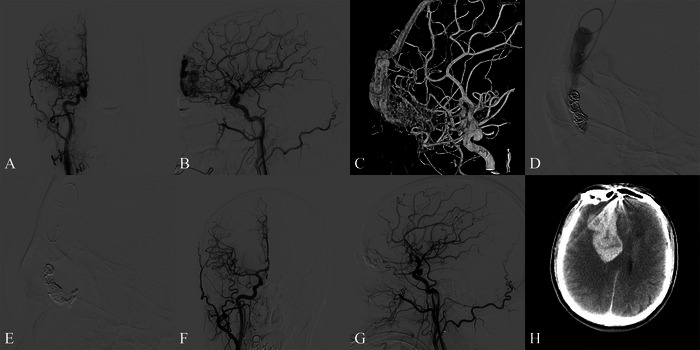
The patient was incidentally diagnosed with an intracranial vascular malformation during routine medical examination. Anteroposterior (A) and lateral (B) views of the right internal carotid artery angiogram revealed a frontal base MPD‐AVM supplied by both the anterior ethmoidal artery and the orbitofrontal artery. 3D reconstruction clearly demonstrated the nidus formation with tortuous and dilated draining veins (C). Transvenous embolization was performed, with microcatheter navigation through the superior sagittal sinus to access cortical veins, followed by deployment of two coils (D). Using the retrograde pressure cooker technique (RPCT), 1.2 mL of Onyx was injected (E). Immediate post‐procedure angiograms showed complete AVM obliteration (F, G). The patient subsequently experienced a severe hemorrhagic event, with CT revealing a massive right frontal lobe hematoma accompanied by contrast extravasation (H).
